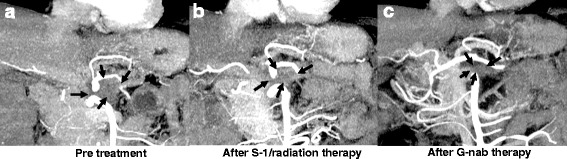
Coronal computed tomography images. a Before chemoradiation. b After chemoradiation. c After gemcitabine with nab-paclitaxel therapy. There are no significant differences in tumor volume between a and b, but c demonstrates tumor shrinkage to 1.2 cm
